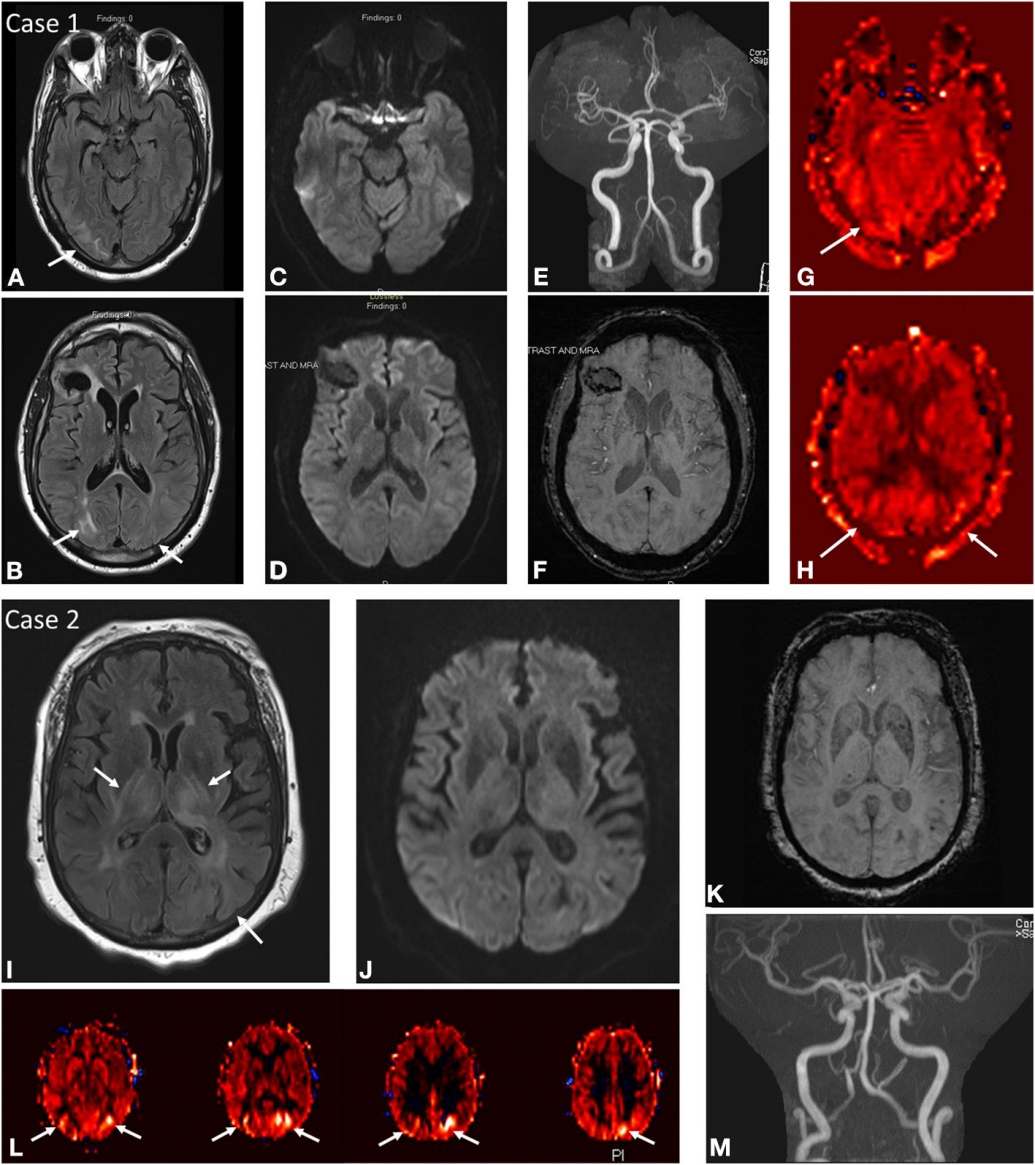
Case 1: A 45-year-old male brought in by ambulance after being found down with seizure activity in the setting of positive urine toxicology screen for cocaine and methamphetamines as well as severe hypertension of 235/101. Past medical history notable HIV on HAART, chronic Hepatitis C, ESRD on HD, and remote right frontal hemorrhage. MRI brain at initial presentation demonstrates abnormal FLAIR hyperintensity (A,B) in the subcortical white matter of the right greater than left occipital lobes with corresponding mixed ASL signal hyperintensity and hypointensity in these regions (G,H). No DWI (C,D) or SWI (F) signal abnormality aside from remote right frontal hemorrhage. No focal arterial high-grade stenosis or occlusion on MR angiography (E). Case 2: A 68-year-old female presenting with seizure activity in setting of past medical history notable for CKD, DM, HTN, HLD, CHF, and COPD. MRI brain at initial presentation demonstrates abnormal FLAIR hyperintensity (I) in the bilateral thalami, bilateral posterior limbs of the internal capsules, bilateral external capsules, and left putamen with more subtle signal hyperintensity in the subcortical white matter of the left occipital lobe. Corresponding ASL signal hyperintensity (L) in the left greater than right occipital lobes. No associated DWI (J) or SWI (K) signal abnormality in these regions, although the patient was noted to have a punctate acute infarct in the right temporo-occipital region (not shown). No focal arterial high-grade stenosis or occlusion on MR angiography (M).
